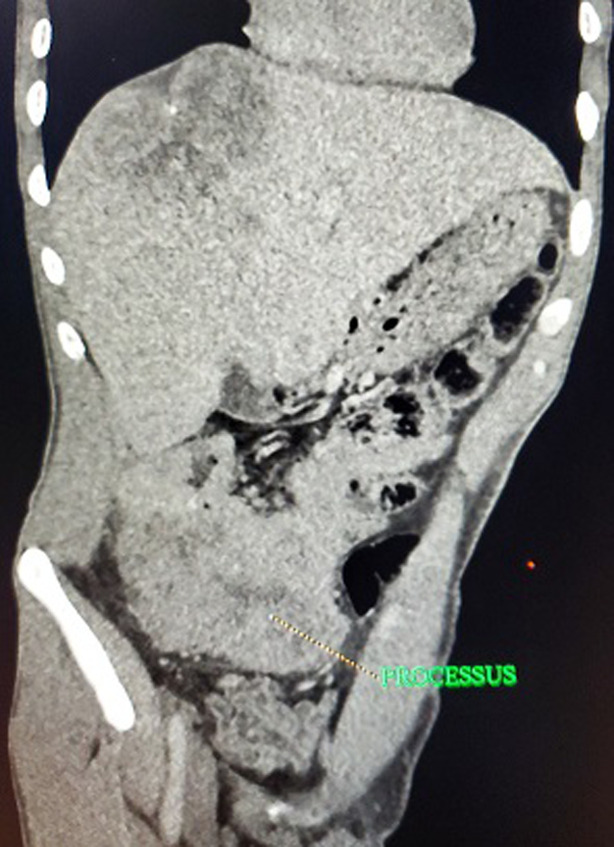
preoperative computed tomography scan, showed a large mass of polylobed appearance of the coecum extended to the colonic angle, associated with hepatic lesion
